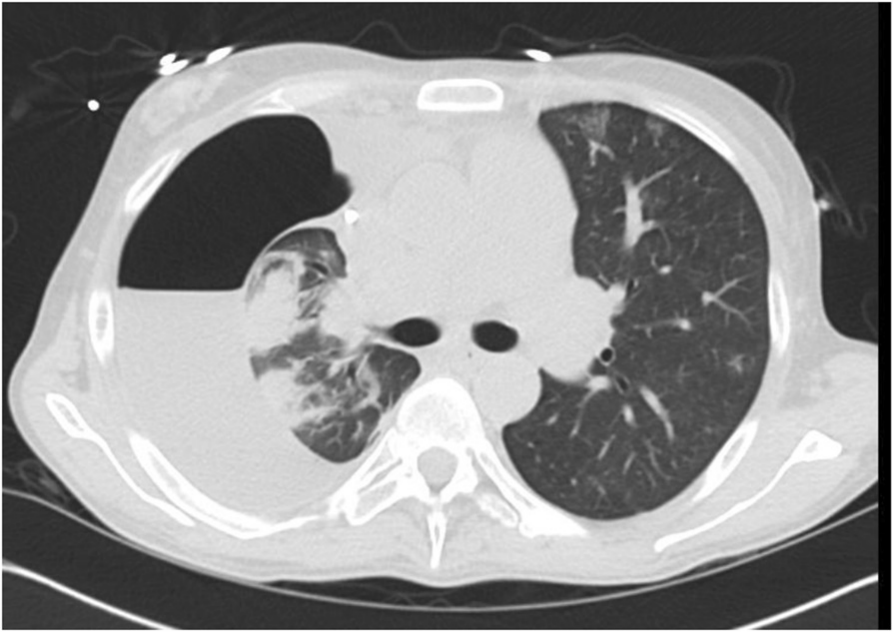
Chest CT post-OLT: Large right-sided hydropneumothorax with underlying visceral and parietal pleural thickening and fibrosis.
